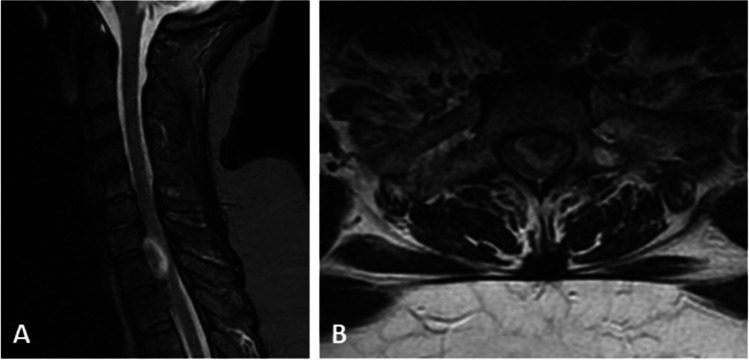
MRI (a sagittal sequence on STIR; b axial sequence on T2) showed intradural extramedullary lesion located ventrally and on the left side with severe spinal cord compression at the level of C7-T1
